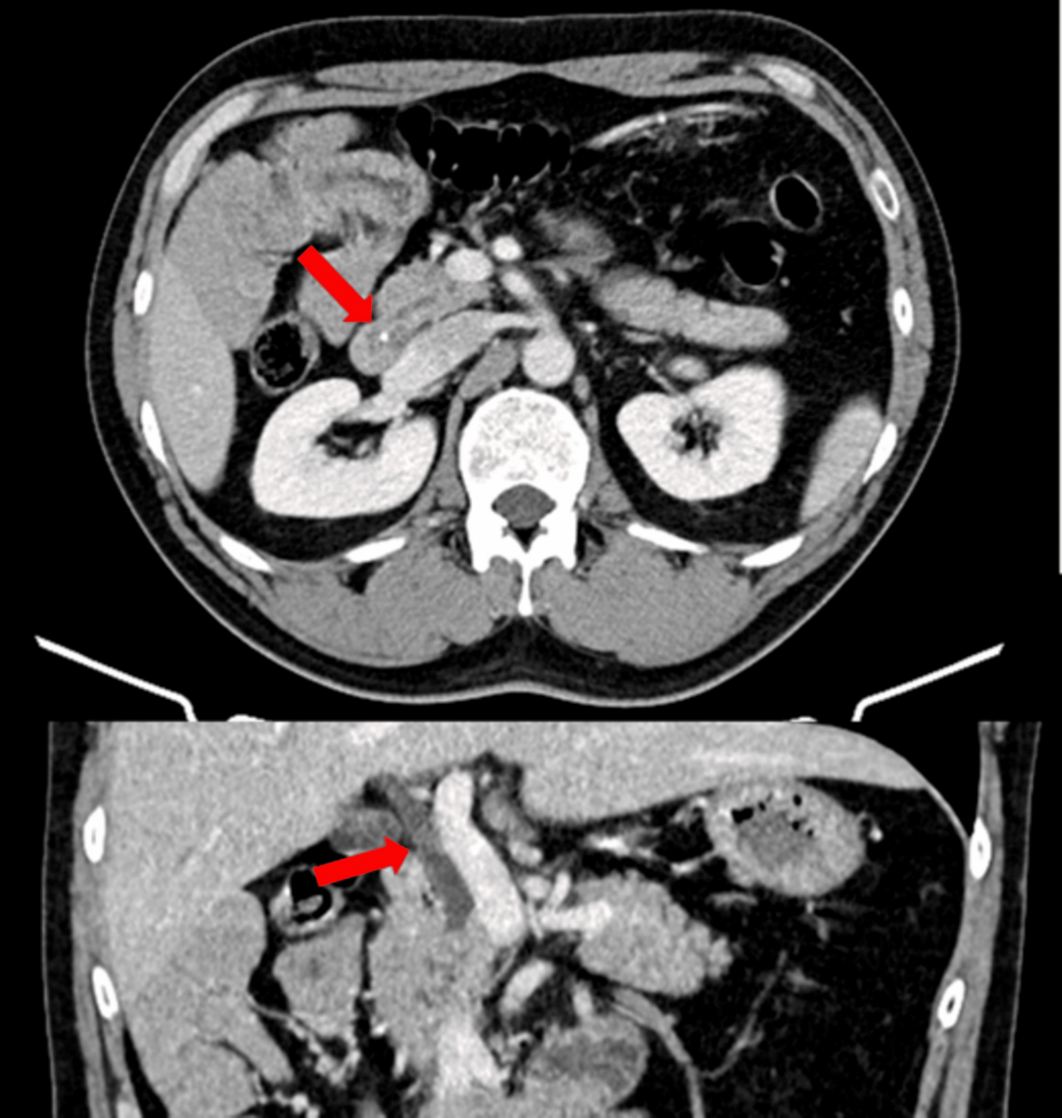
CT findings in Case 1 Abdominal CT showing gallbladder enlargement and wall thickening, consistent with acute calculous cholecystitis (red arrow).
